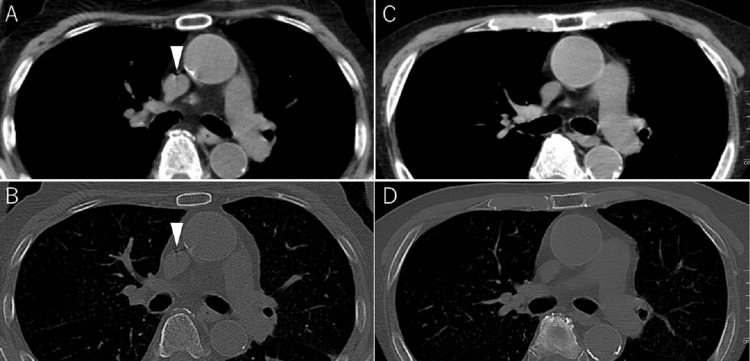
Air in the superior vena cava on subsequent chest CT and its later disappearance. (A) Axial chest CT image (soft tissue window) showing a small focus of air within the superior vena cava (arrowhead). (B) Corresponding bone window image confirming the same finding (arrowhead). (C, D) Follow-up CT images at the same level, showing later disappearance of the air.
